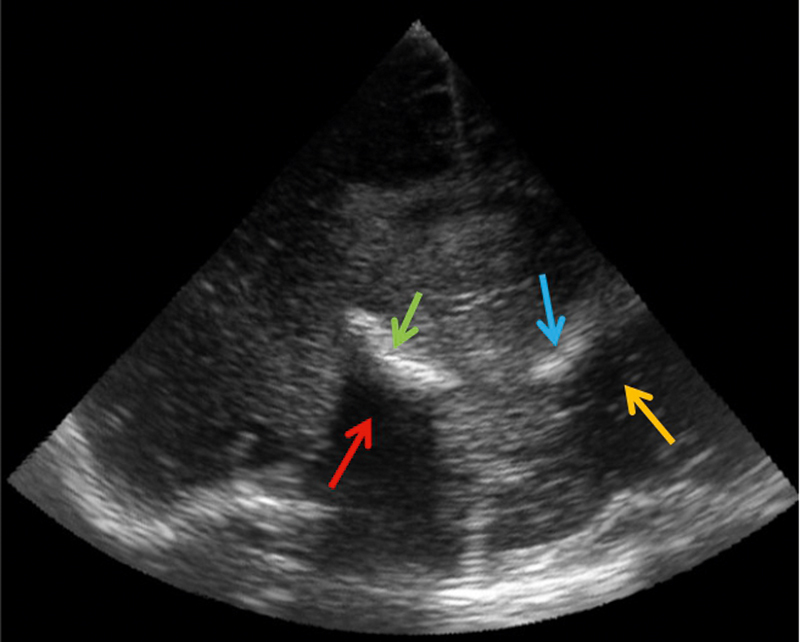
B-mode ultrasound image of a high-grade glioma showing
